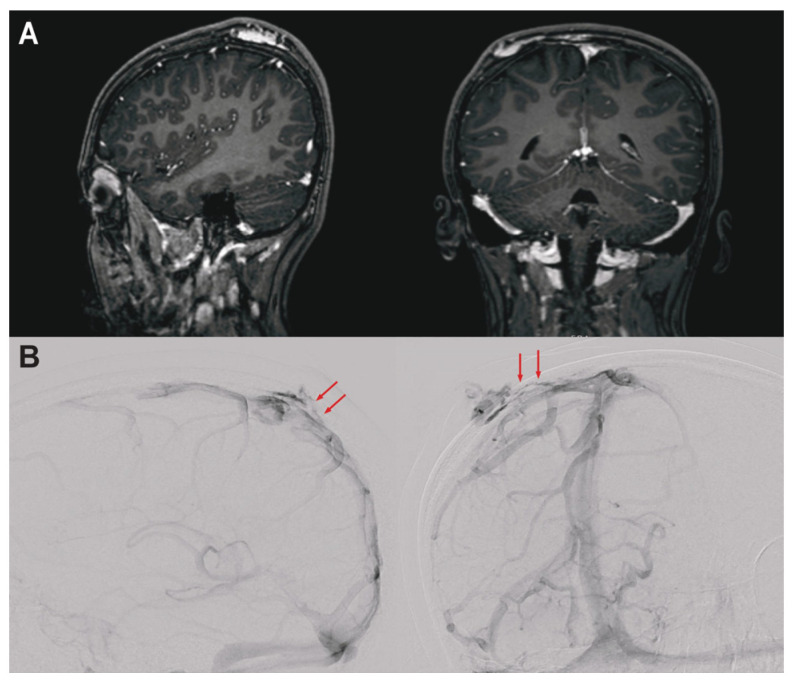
Pre-operative neuroimaging studies for a 16-year-old female with low-flow venous malformation. (A) Sagittal (left) and coronal (right) post-contrast T1-weighted MRI shows well-defined tortuous vessels in the right parietal scalp, suspicious for a venous malformation. The enhancement in the parietal bone overlying the superior sagittal sinus, in combination with subtle transdiploic tracks of linear enhancement, raised concern for patency of venous channels and a sinus pericranii. (B) Lateral and right anterior oblique digital subtraction angiography images following right internal carotid artery injection confirm a slow-flow venous lesion with modest blush and without evidence of arteriovenous shunting. The transcalvarial connections are apparent on both images (red arrows).
